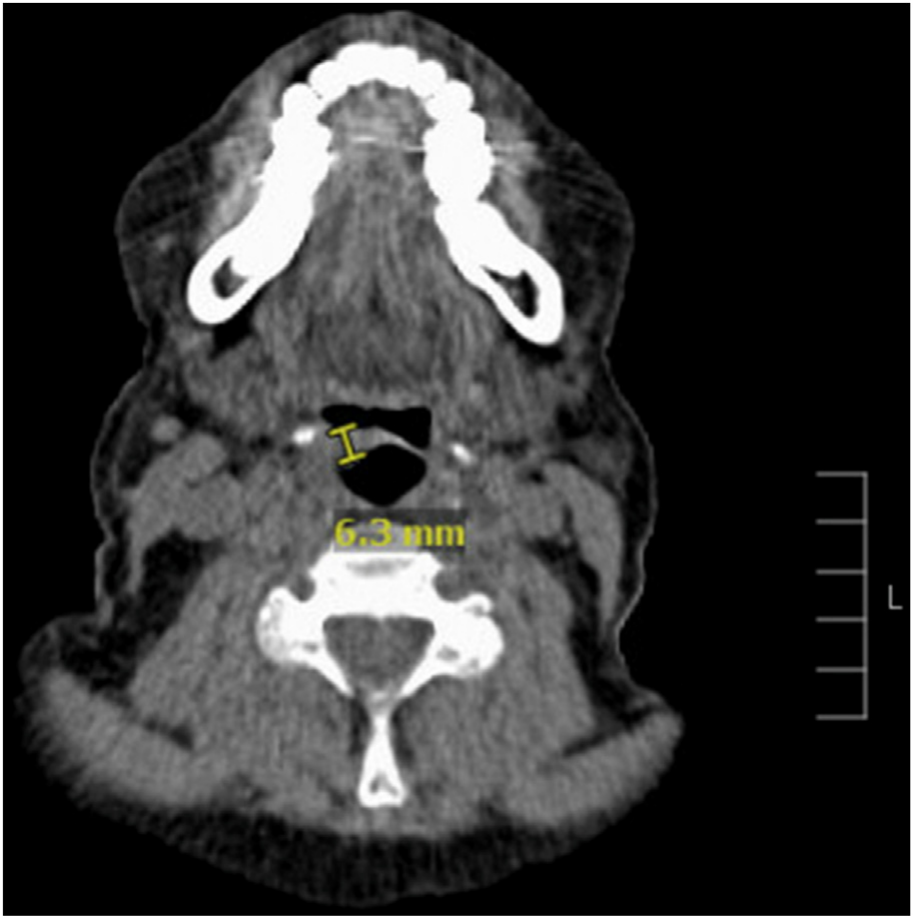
Axial computed tomography scan of oropharynx demonstrating a well-defined supraglottic mass (*yellow marker*) 6.3 millimeters in width, revealed to be epiglottic involvement of mycosis fungoides.
